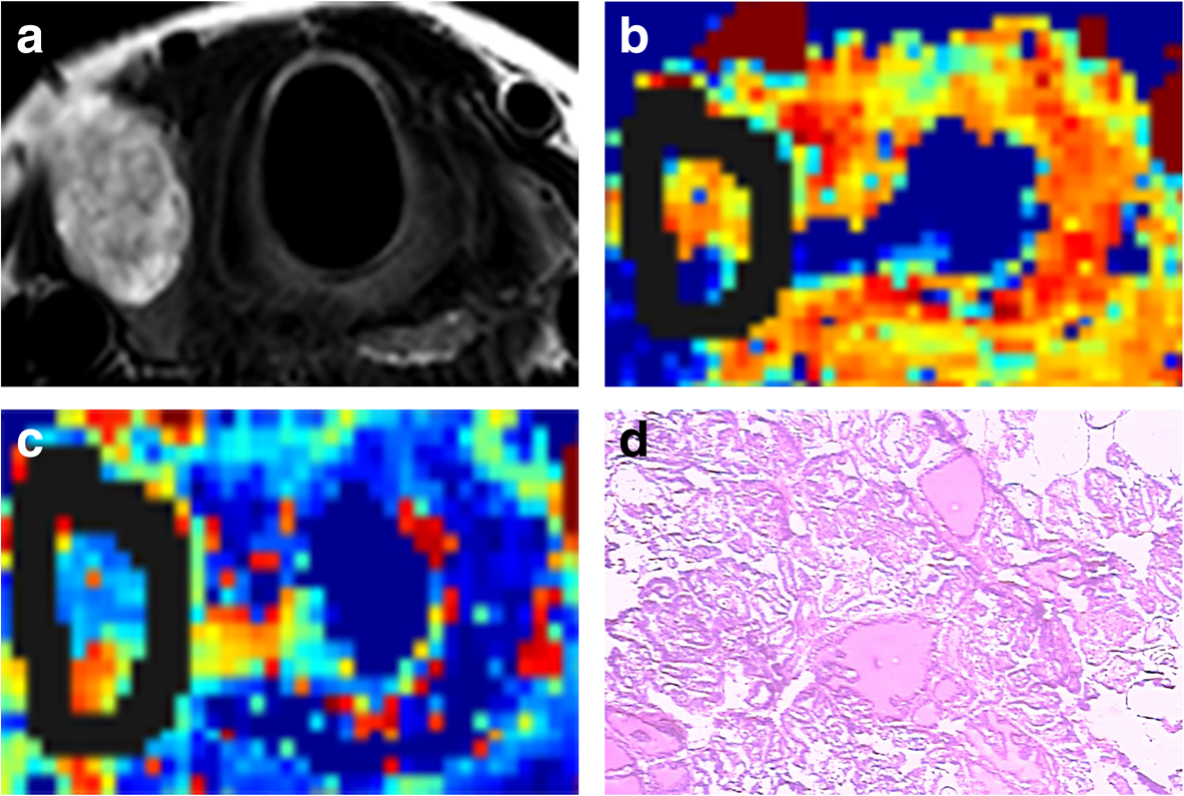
Images of a 65-year-old woman with right lobe papillary thyroid cancer (eccentric part containing cystic necrosis). a. Axial T2-weighted image. b-c. IVIM colour map (*D* and *f*). *D* = 0.83 × 10^− 3^ mm^2^/s, *f* = 32.15%. Solid lines show borders containing cystic necrosis. d. Nuclei arranged closely in papillary thyroid carcinoma (HE, × 100)
